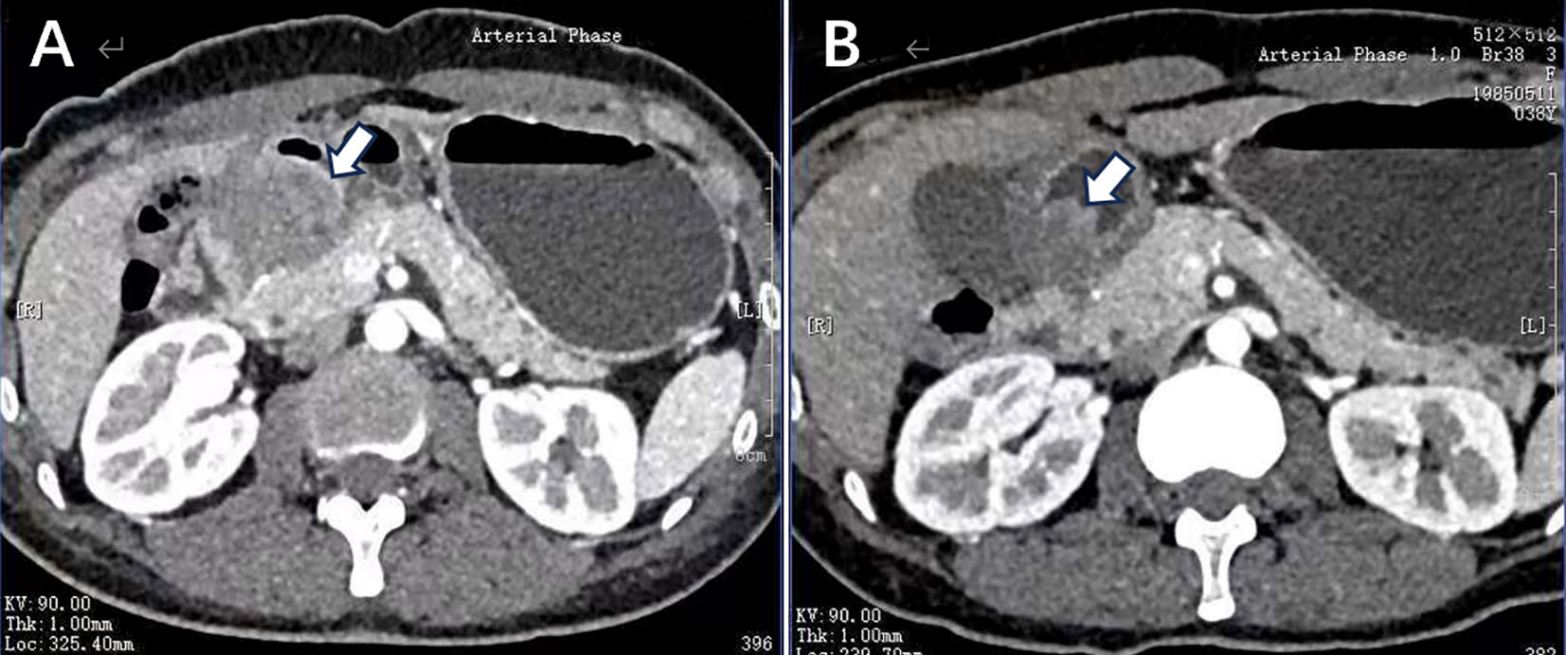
(A) Before systemic therapy, enhanced CT image showed edema of the gastric antrum, with a lesion located between the gastric antrum and pancreatic head demonstrating heterogeneous enhancement. (B) After the treatment of nivolumab plus XELOX, the follow-up imaging revealed reduced edema in the gastric antrum and a decrease in the size of lesion.
